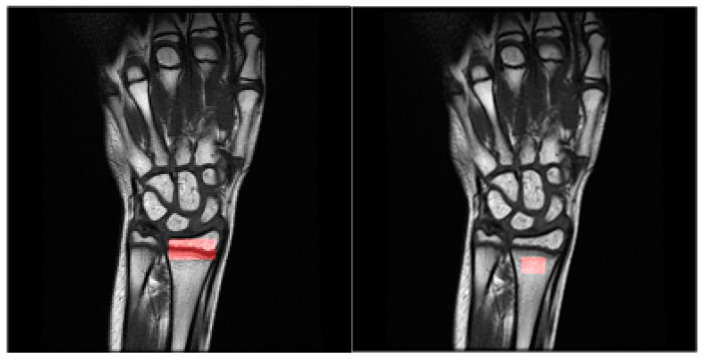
Exemplary magnetic resonance imaging. The red rectangle shows the growth region in the image on the right and bone region on the left scan.
